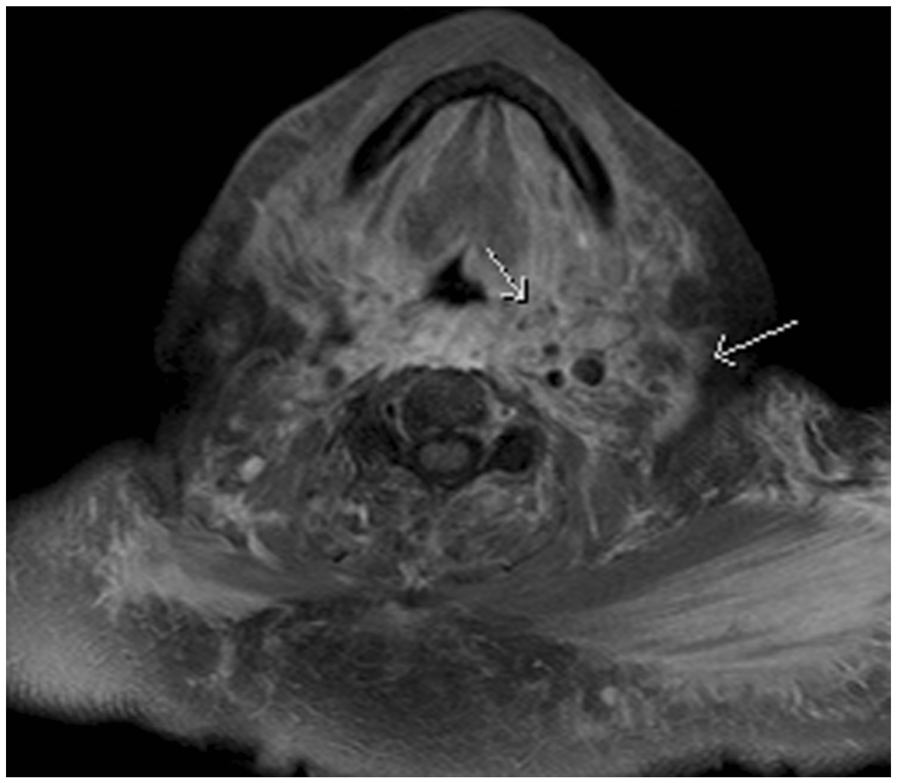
Magnetic resonance imaging of the neck. Magnetic resonance imaging of the neck on day 20 showed diffuse, predominantly left-sided inflammatory infiltration of epi- and mesopharyngeal tissues (arrows).
